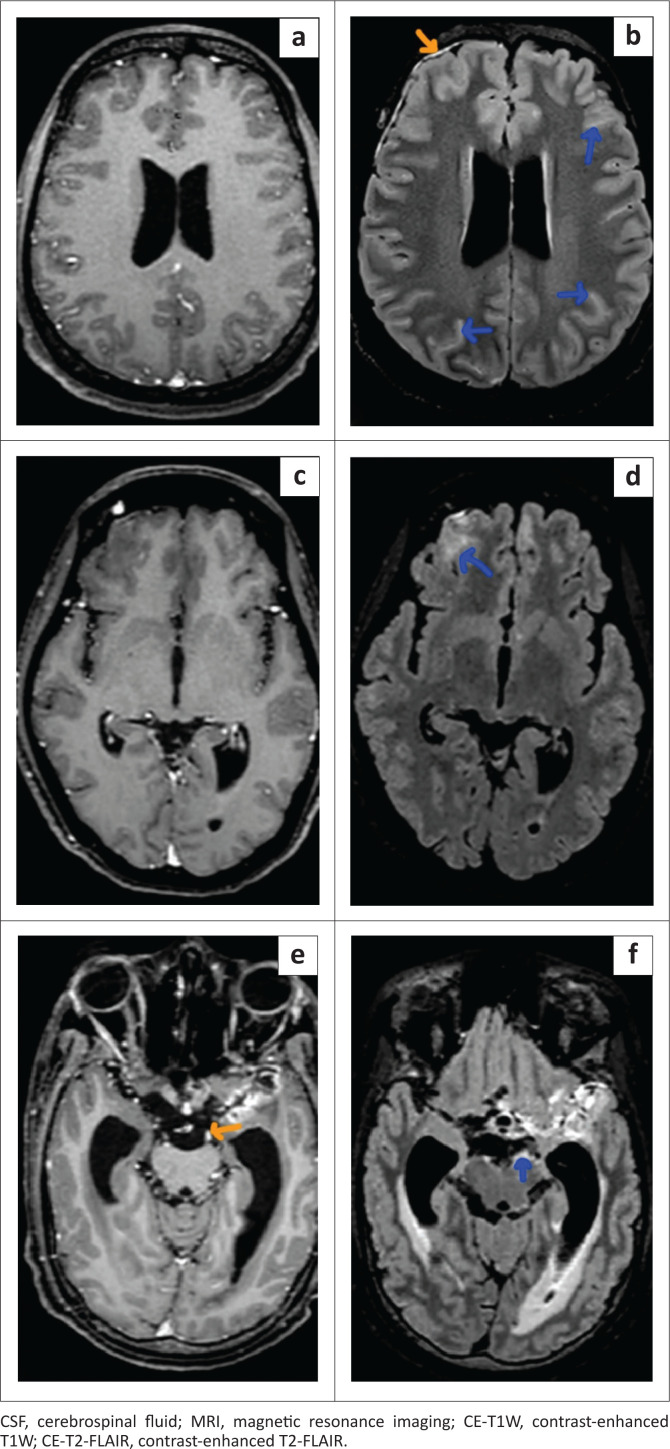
Axial brain MRI of three different patients. (a and b) A 36-year-old male patient with CSF proven meningitis. Thin sulcal enhancement along both cerebral hemispheres (blue arrows) and pachymeningeal enhancement along the right frontal lobe (orange arrow) are seen on the CE-T2-FLAIR (b) and not appreciated on CE-T1W (a). (c and d) A 40-year-old female with CSF proven meningitis. Thin sulcal enhancement along the right frontal lobe (blue arrow) is seen on CE-T2-FLAIR (d) and not appreciated on CE-T1W (c). (e and f) A 35-year-old male patient with CSF proven meningitis. Meningeal enhancement around the midbrain is seen on CE-T2-FLAIR (f) (blue arrow) and not seen in CE-T1W (e) (orange arrow represents a vessel).
